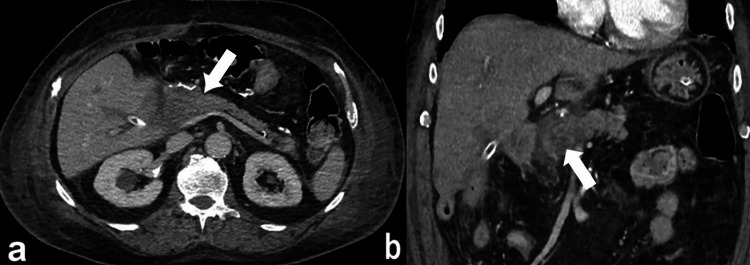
Typical CT findings a, b: Contrast-enhanced multidetector computed tomography (MDCT) scan performed on postoperative day three in a patient following pylorus-preserving pancreaticoduodenectomy due to bile output >300 ml/day from the surgical drain and signs of systemic inflammation. A subtle rim of fluid collection is seen around the bilio-digestive anastomosis (BDA), raising suspicion of an anastomotic insufficiency (white arrow).
